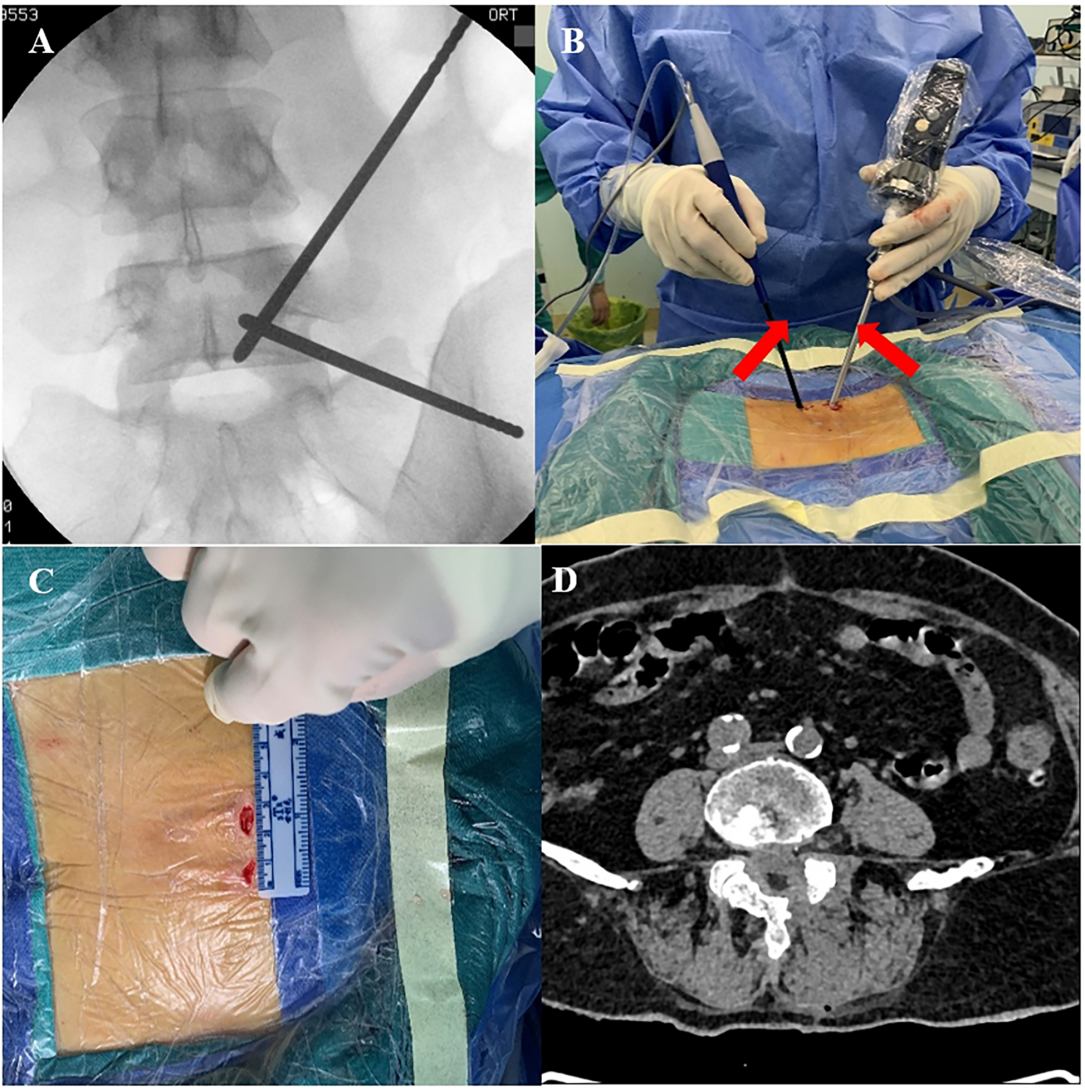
Intraoperative UBE. (A) Preoperative C-arm fluoroscopy to localize the target segment. (B) Manipulation and observation in two portals (red arrows). (C) Length of UBE surgical incision. (D) Postoperative CT showing complete decompression of the spinal canal.
